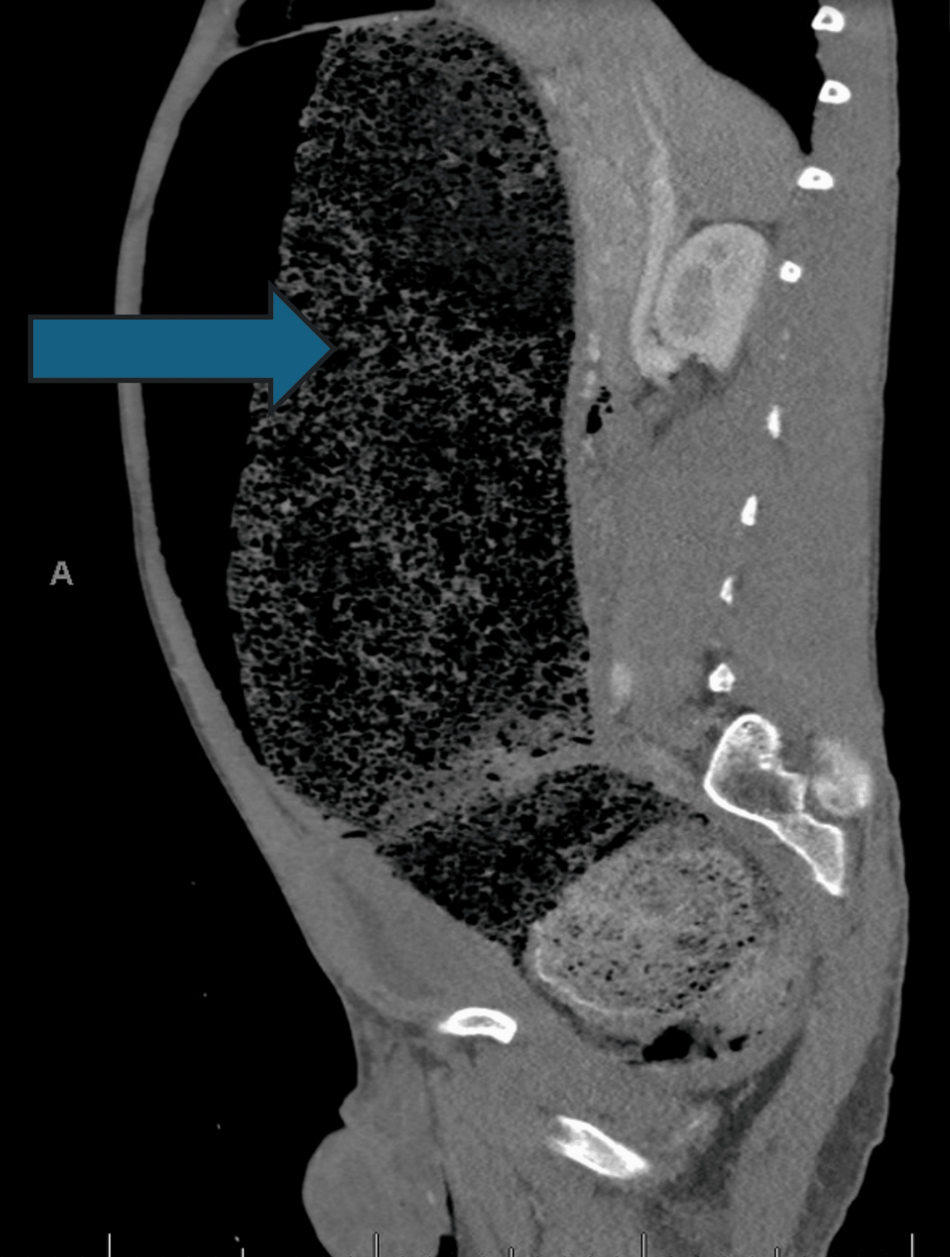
CT sagittal view of abdomen/pelvis showing gigantic fecaloma (blue arrow) with compression of abdominal structures
